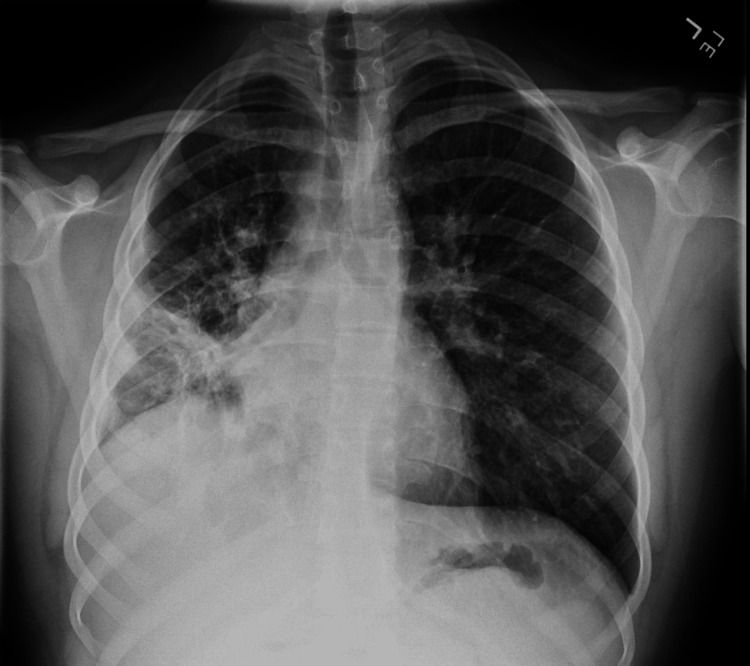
Chest X-rays after two months of treatment Right lung aeration appeared improved. Perihilar and right lower lobe coarsened interstitial markings and multifocal streaky parenchymal opacities were seen. Right-sided pleural fluid or thickening is identified. Left lung was well inflated with some persistent coarsening of interstitial markings and faint patchy alveolar densities.
